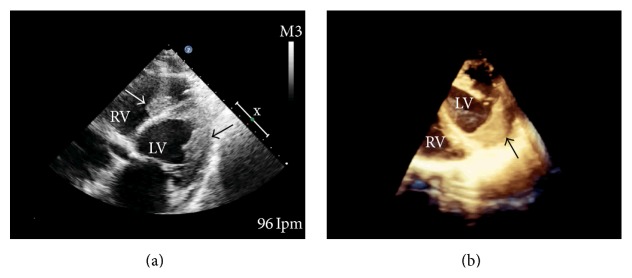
(a) Transthoracic echocardiogram in a modified apical four-chamber view, showing the left ventricular apex with obliteration and the lateral wall thickened by an image suggestive of a thrombus (black arrow) in the right ventricle and image suggestive of a smaller thrombus attached to the septum (white arrow). (b) Transesophageal echocardiogram with a 3D reconstruction, showing a thrombus in the left ventricle (black arrow). LV = left ventricle; RV = right ventricle.
